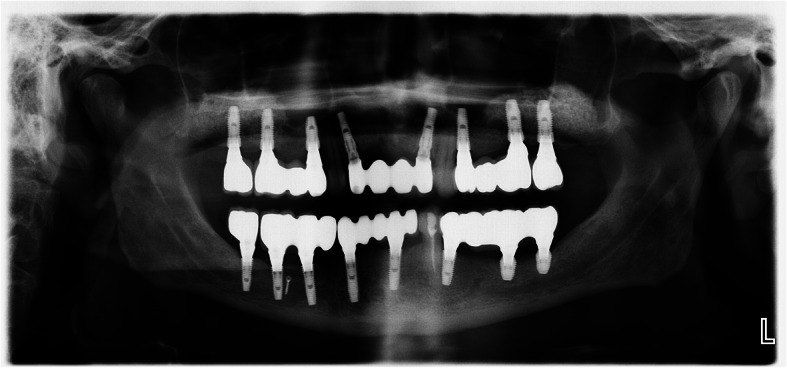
A panoramic radiography after the delivery of the final prosthesis
